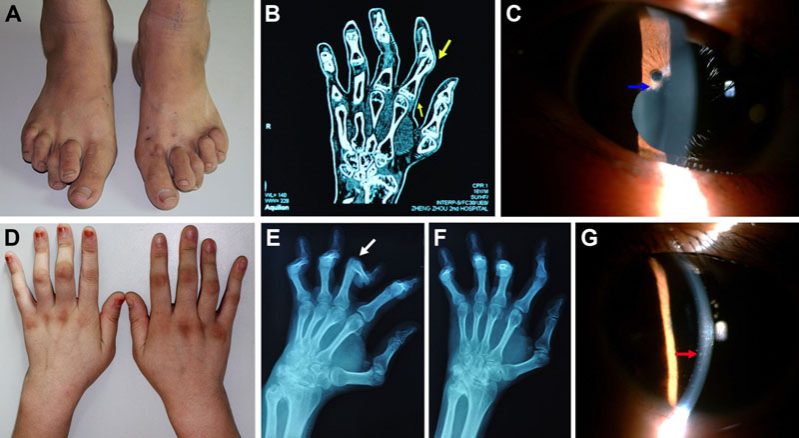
Representative photographs of arthritis and uveitis of patients in the family. A: General appearance showed deformities of the feet in patient (III:6). B: The coronal reconstruction section of CT scan of patient (III:6) revealed erosion of bone at the edges of the joint in the MCP joints, joint space narrowing and subluxation in the proximal interphalangeal (PIP) joints (yellow arrow). C: The right eye of the patient (III:6) revealed partial posterior synechia (blue arrow). D: Deformity of the hands of the proband (III:5). E and F: X-rays of patient (II:5) showed multiple and symmetric joint involvement, generalized osteoporosis, joint space narrowing, poorly defined edges of the articular surfer, subluxation, contracture and ankylosis of the PIP joints (white arrow). G: the right eye of the proband (III:5) showed dot-like calcific keratopathy in Bowman's membrane and the cornea between the opacities remained clear (red arrow).
